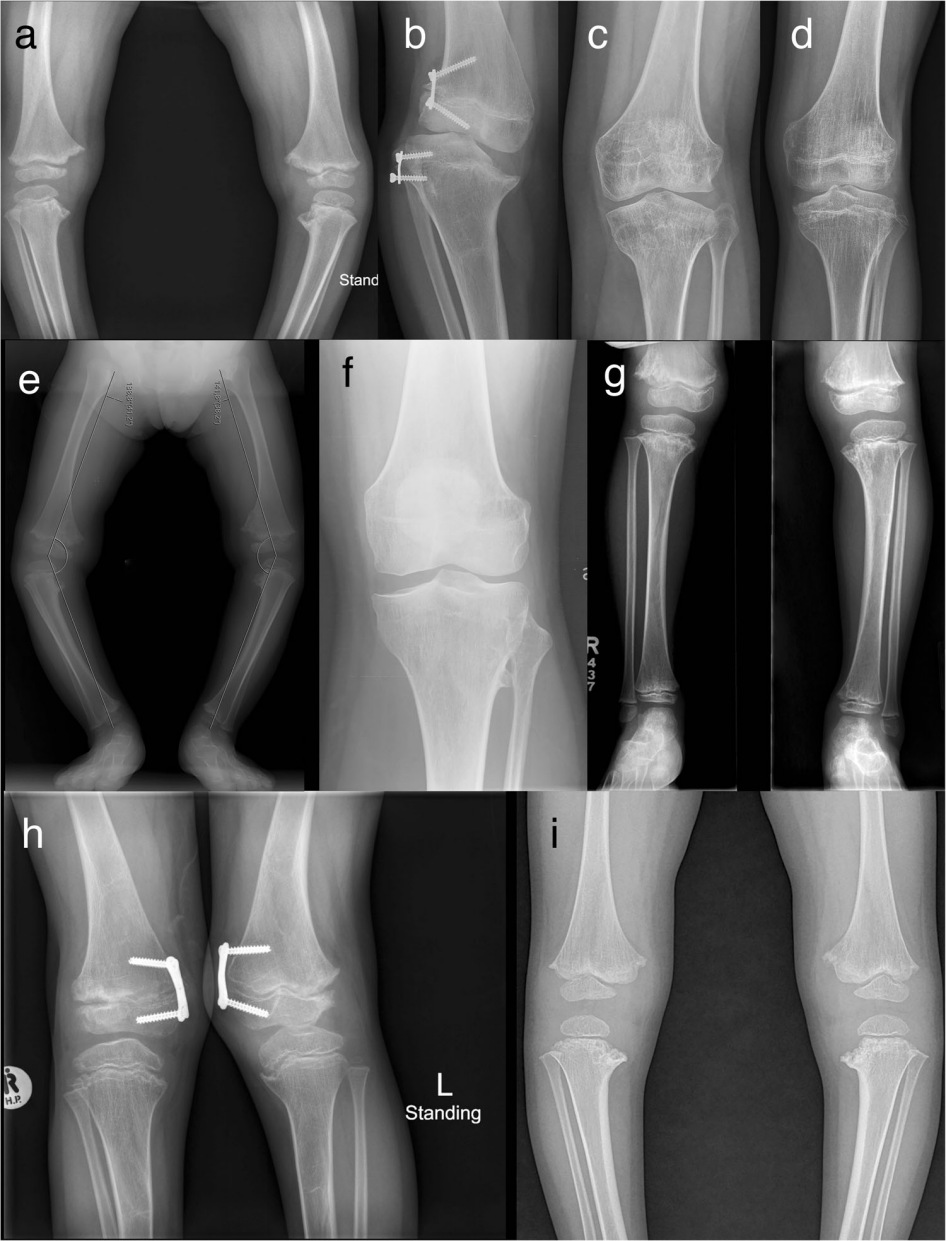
Knee radiographs. a Patient 1-III:4, radiograph at age 3 years; showing irregular metaphyses and small epiphyses of the distal femora and proximal tibiae with mild *genu varum*. b Patient 3, radiograph at age 11 years; showing *genu varum* and defective ossification of the medial aspect of the proximal tibial epiphysis. 8-plate guided growth was inserted to restore the knee deformity. c Patient 4-II:1 radiograph at age 18 years; showing mild epiphyseal dysplasia. d Patient 4-II:4, radiograph at age 15 years; showing mild epiphyseal dysplasia. e Patient 5-III:1, radiograph at age 3 years; showing small epiphyses and metaphyseal irregularities of the distal femora and proximal tibiae with bilateral *genu varum*. f Patient 5-II:2, radiograph at age 42 years; showing premature degenerative joint disease. g Patient 6-II:1, radiograph at 7 years; showing metaphyseal changes of the knees and mild *genu varum*. h Patient 7-III:2, radiograph at 7 years; showing mild metaphyseal changes and mildly flat epiphyses of the knees, *genu valgum*. 8-plate guided growth surgery partially restored severe *genu valgum*. i Patient 7-III:3, radiograph at 2 years; showing mild metaphyseal changes, mildly flat epiphyses of the knees and *genu varum*.
